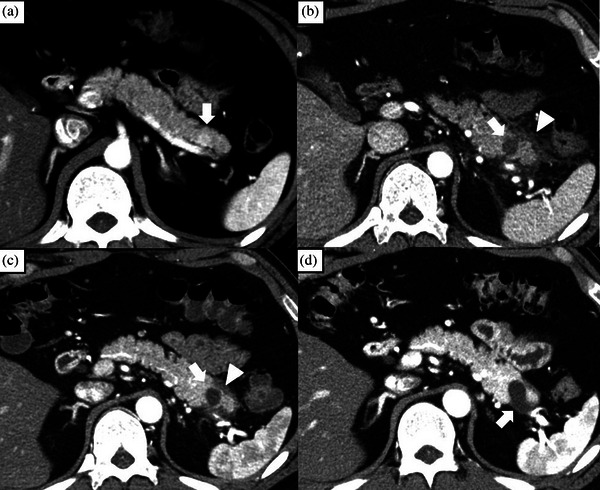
Patients with acute pancreatitis after EUS‐guided radiofrequency ablation (Patient number 5). (a): An enhanced tumor measuring 10 mm located in the tail of the pancreas on CE‐CT (arrow). (b): CE‐CT performed 3 days after the procedure revealed that the tumor site was ablated (arrow) and inflammatory findings had spread beyond the pancreas (arrowhead). (c): CE‐CT scan at 1 month showed that the enhancement of the tumor had disappeared (arrow), and the peripancreatic inflammation had almost resolved (arrowhead). (d): CE‐CT at 6 months reveals the formation of a pseudocyst at the ablation site (arrow). CE‐CT, contrast enhanced‐computed tomography; EUS, endoscopic ultrasonography.
